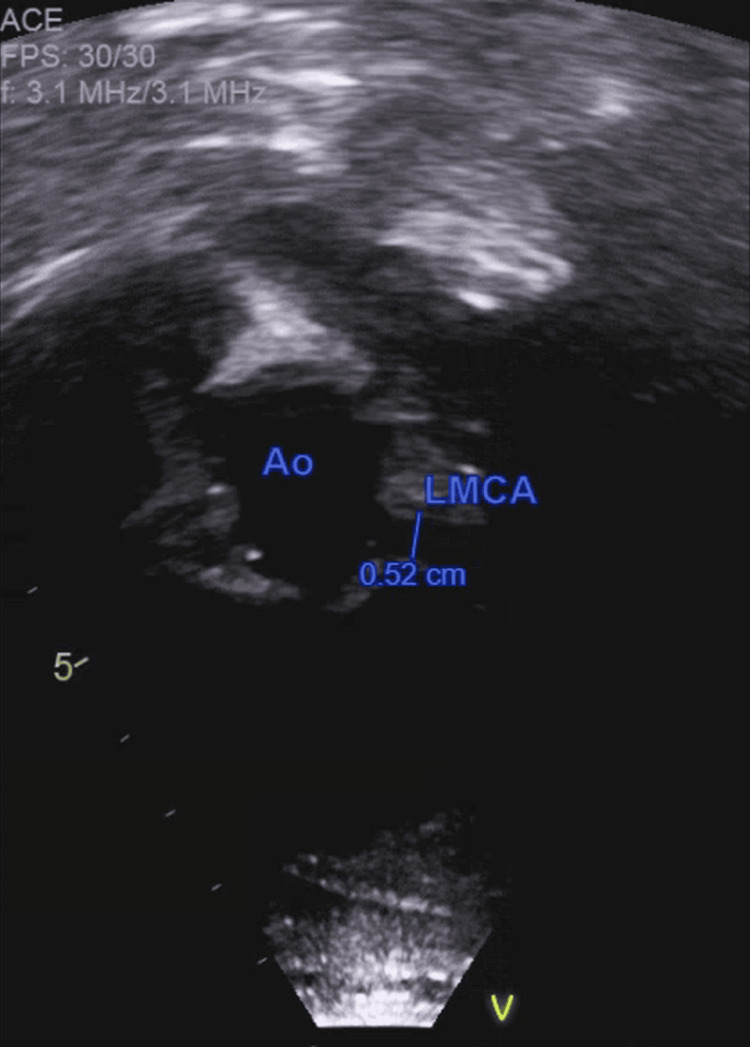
Transthoracic echocardiogram demonstrating dilation of the LMCA origin Parasternal short-axis view at the level of the aortic root showing the LMCA arising from the left coronary sinus. The measured diameter of the LMCA was 0.52 cm, corresponding to a Z-score of 4.2, consistent with coronary dilation. LMCA, left main coronary artery
